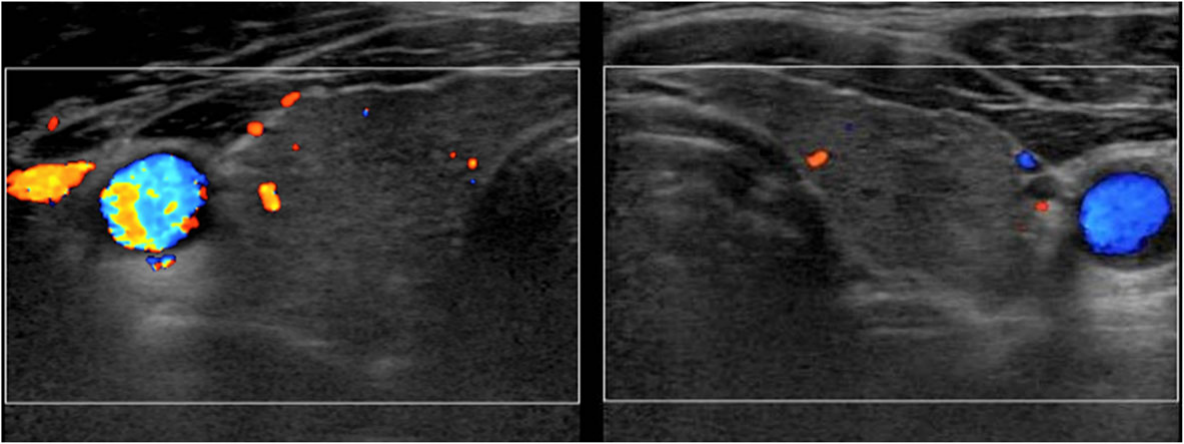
Thyroid ultrasonography findings. Hypoechoic regions are scattered throughout the thyroid gland. No increase in blood flow in the thyroid gland was observed
